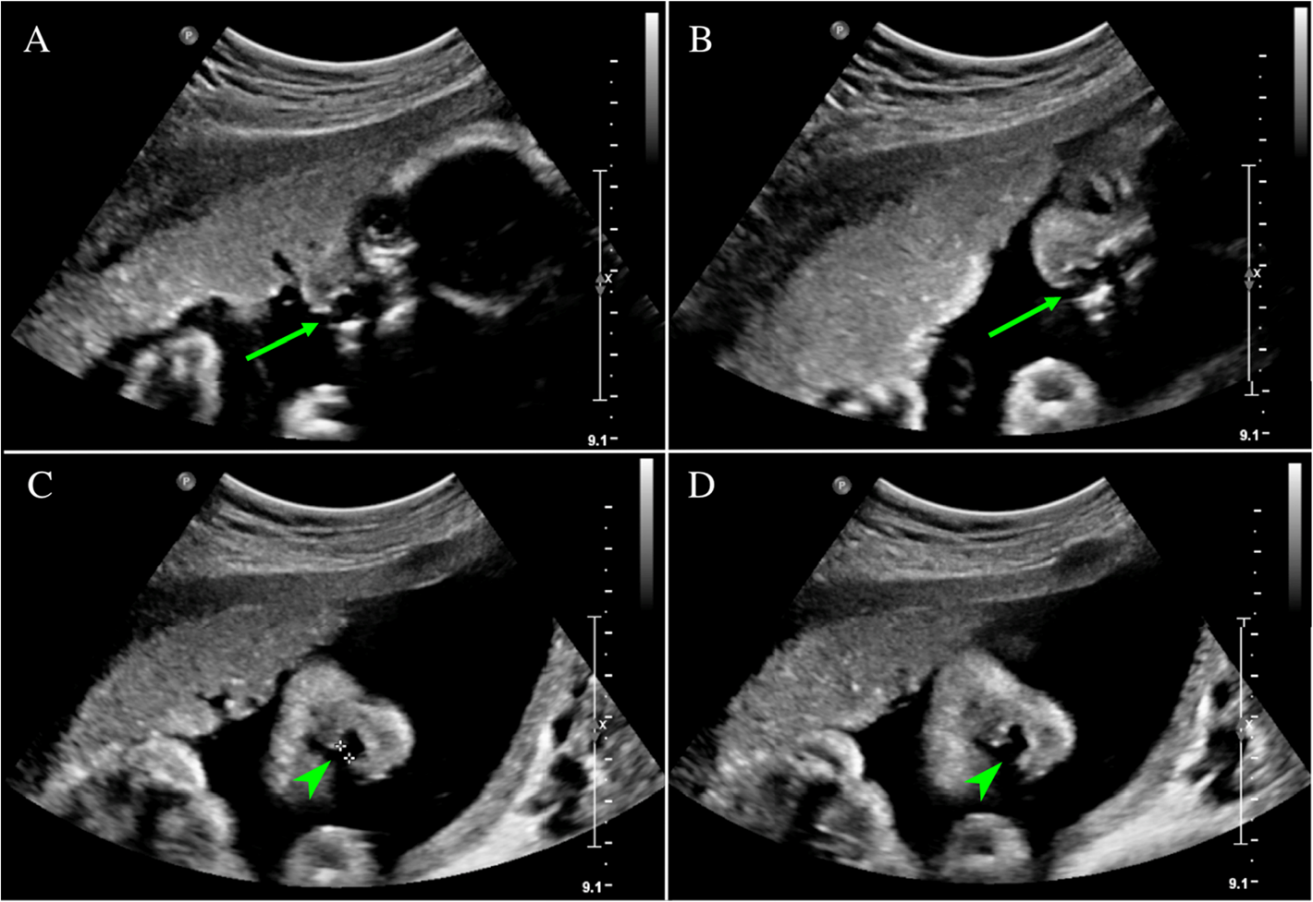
Transabdominal ultrasonography of Case 1, 26 weeks’ gestation. a, b: Arrow indicates the cleft palate site. c, d: Arrowhead indicates the cleft lip site
